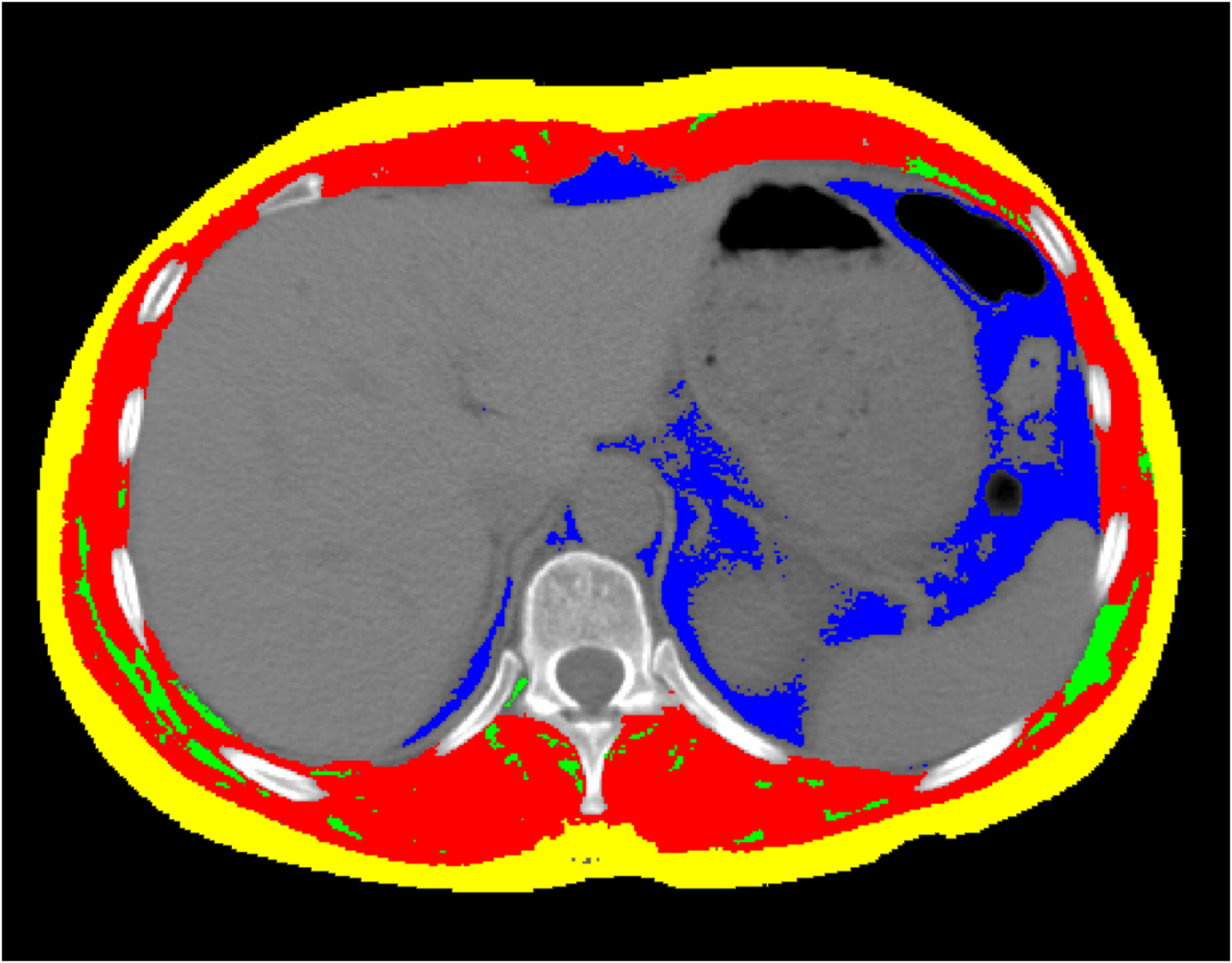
The CT image of the 11th thoracic vertebral plane. Images of cross-sectional area of skeletal muscle (Red colored area) with the Hounsfield unit (HU) from − 29 to + 150, and of intermuscular fat tissue (Green colored area) from − 190 to − 30, and of subcutaneous fat tissue (Yellow colored area) from − 190 to − 30, and of visceral fat tissue (Blue colored area) from − 150 to − 50, using the SliceOmatic Software version 5.0 (Tomovision, Montreal, QC, Canada). The colored regions are the regions of interest.
